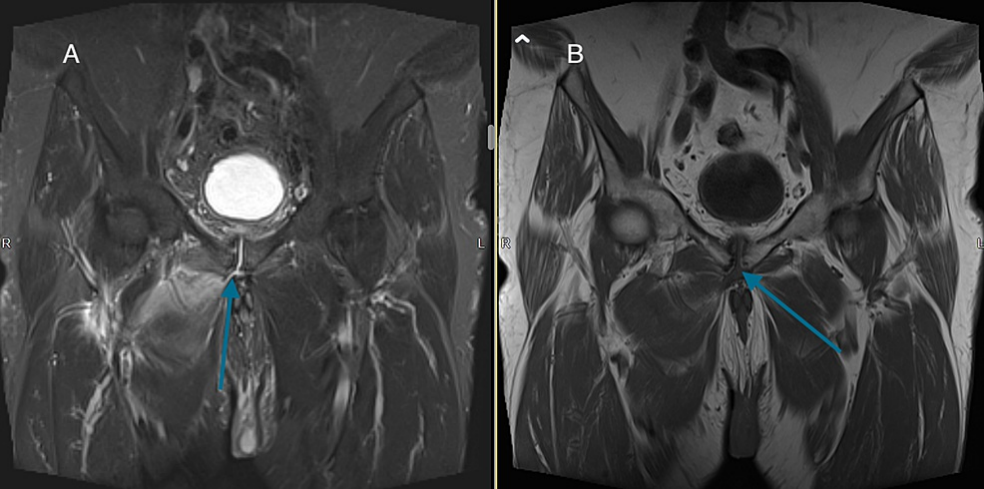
Coronal views: (A) turbo inversion recovery magnitude (TIRM) and (B) T1-weighted image with fluid descending from the symphysis to the right adductor compartment (arrows).
